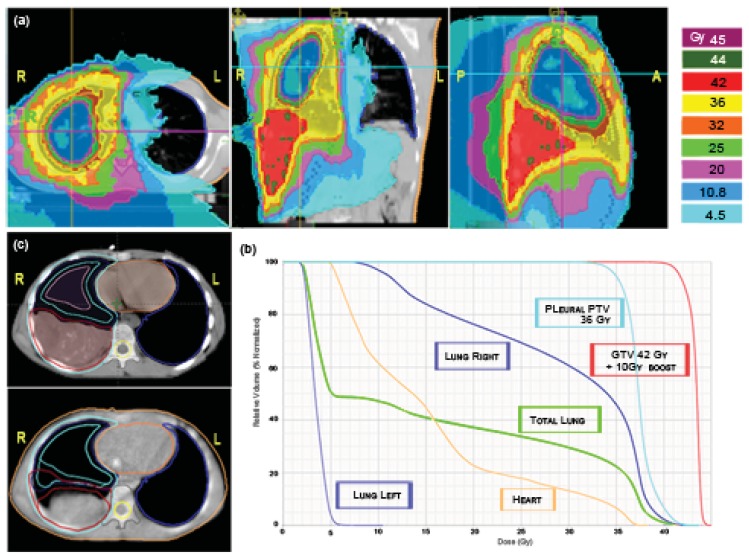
A 10-year-old male affected by right pleural PNET. HT allows different dose gradients between the pleural cavity (36 Gy/20 fractions) (a) and residual disease (42 Gy/20fractions) (b). DVHs of the first phase of treatment for the residual GTV, right pleural PTV, right lung, left lung, total lung volume and heart are shown (c). Further 10 Gy/5 fractions were delivered only to the shrinking tumor (bottom left) after the first phase of treatment on the basis of MV-CT acquisition.
